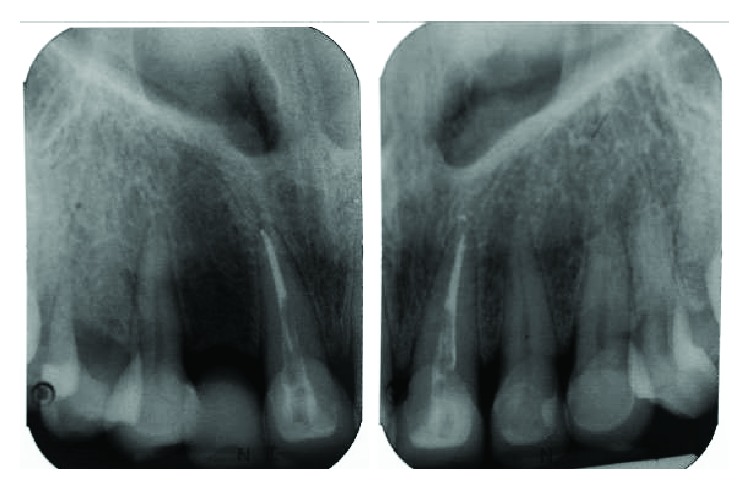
Periapical radiographs of the upper anterior teeth at review visits showing no periapical change or sign of root resorption.
